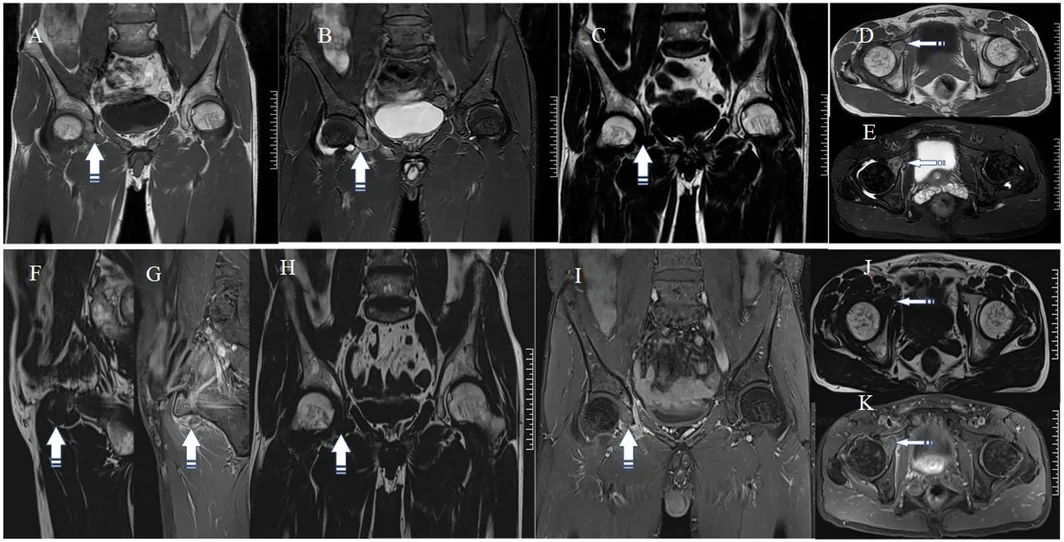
Magnetic resonance imaging of both hips. (A–E) Non‐contrast MRI, and (F–K) Contrast‐enhanced MRI. Mild bilateral hip joint effusion is present, more pronounced on the right, with synovial thickening. A patchy intra‐articular synovial lesion in the right hip demonstrates heterogeneous low‐to‐intermediate signal intensity on T1‐weighted images, heterogeneous signal intensity on T2‐weighted images, and heterogeneous enhancement after contrast administration. The lesion measures approximately 27 × 35 × 25 mm. Thinning of the adjacent anterior acetabular column and mild swelling of the right obturator externus muscle are also present. Arrows indicate the lesion.
